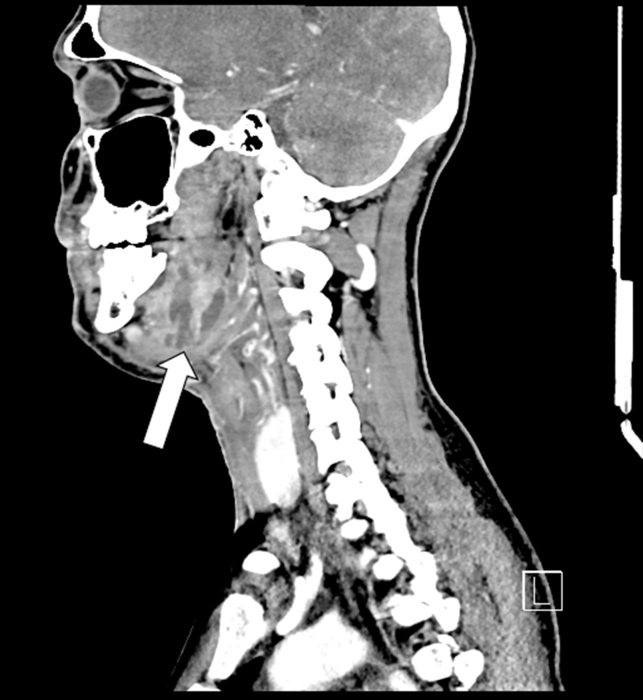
Coronal contrast-enhanced computed tomography of the neck revealing a 4.7 cm multiloculated fluid collection (arrow) within the left submandibular space, with surrounding edema, inflammation, and gas collection.
